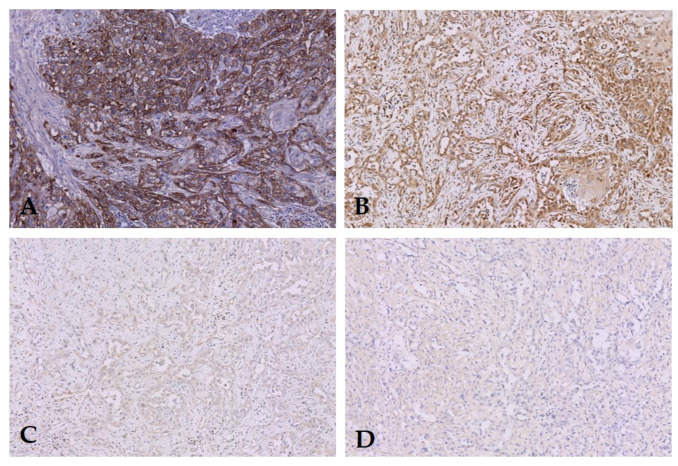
Lymph node metastasis of one patient with expression of (A) EGFR; (B) VEGF; no expression of (C) PSMA and (D) EpCAM. Original magnification 200×.
